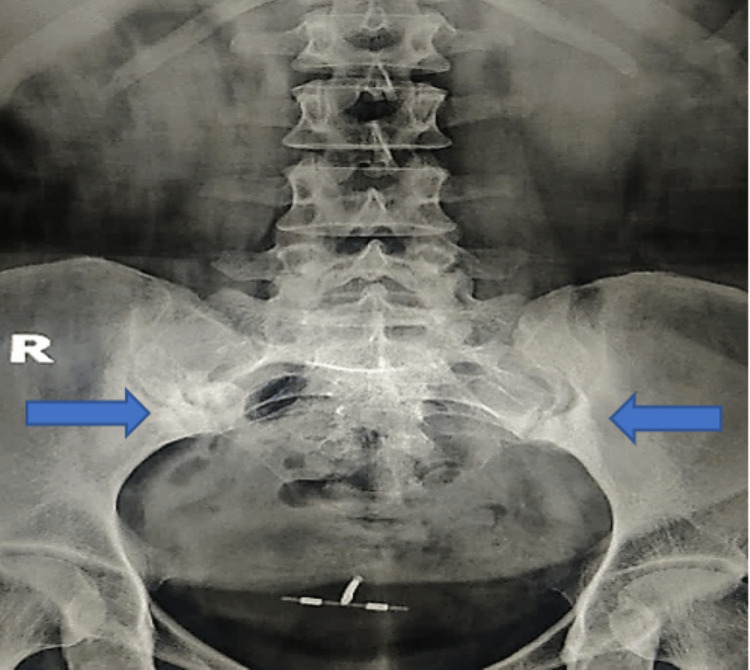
Antero-posterior view of the plain radiograph of pelvis showing triangular area of significant sclerosis over the inferior aspect of iliac border of both the sacroiliac joints.
